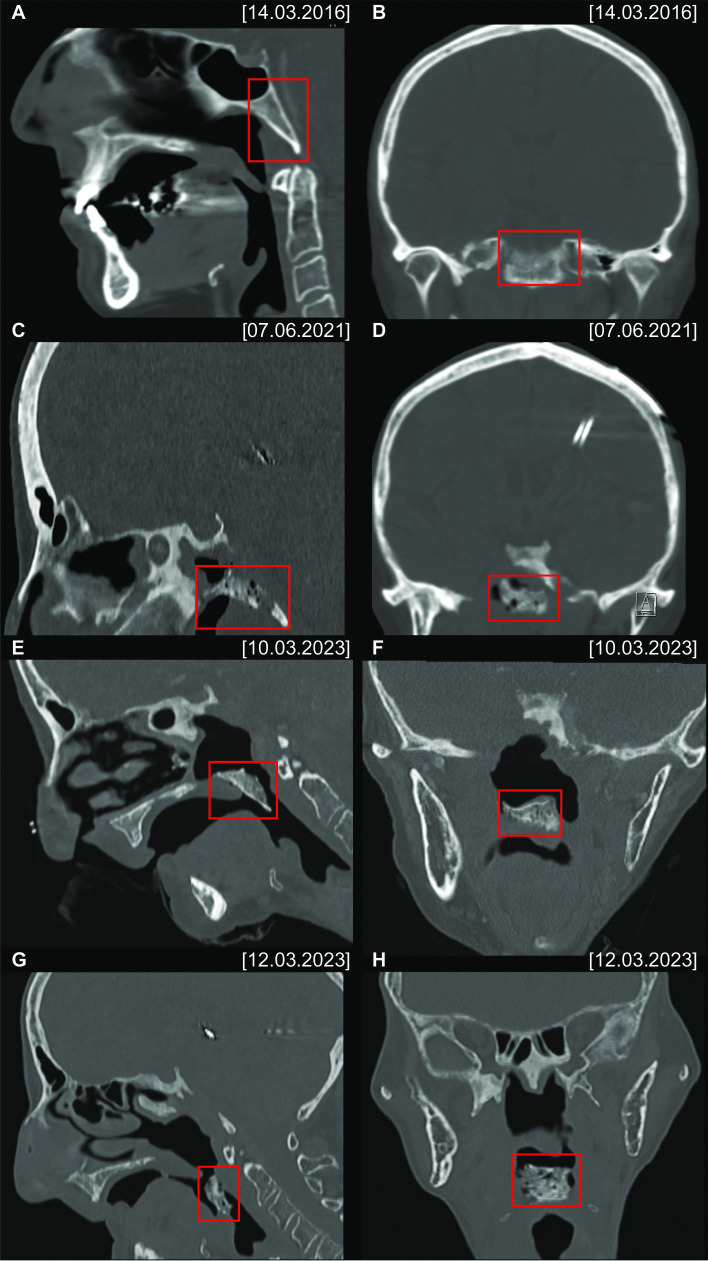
CT scans in the bone window (sagittal: A, C, E, G; coronal: B, D, F, H) show severe radio osteonecrosis of the anterior skull base with a chip fracture of the clivus (red rectangle) and the progredient dislocation
